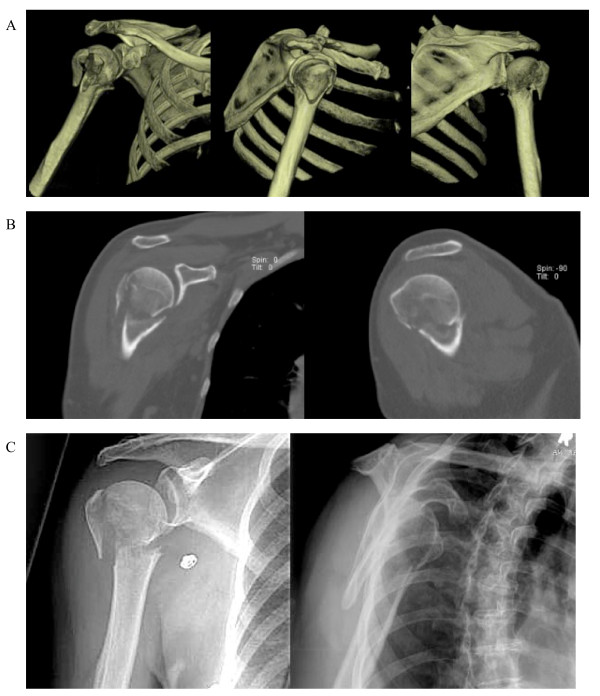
An example of a (A) 3D reconstruction showing AP, lateral, and PA views of a right shoulder. Most popular answers: 50% of raters classified this image as a 3-part fracture (37.5% classified 4-part) and 75% recommended ORIF. From the same patient are (B) coronal and axial views of the CT scan with (C) AP and lateral X-rays.
